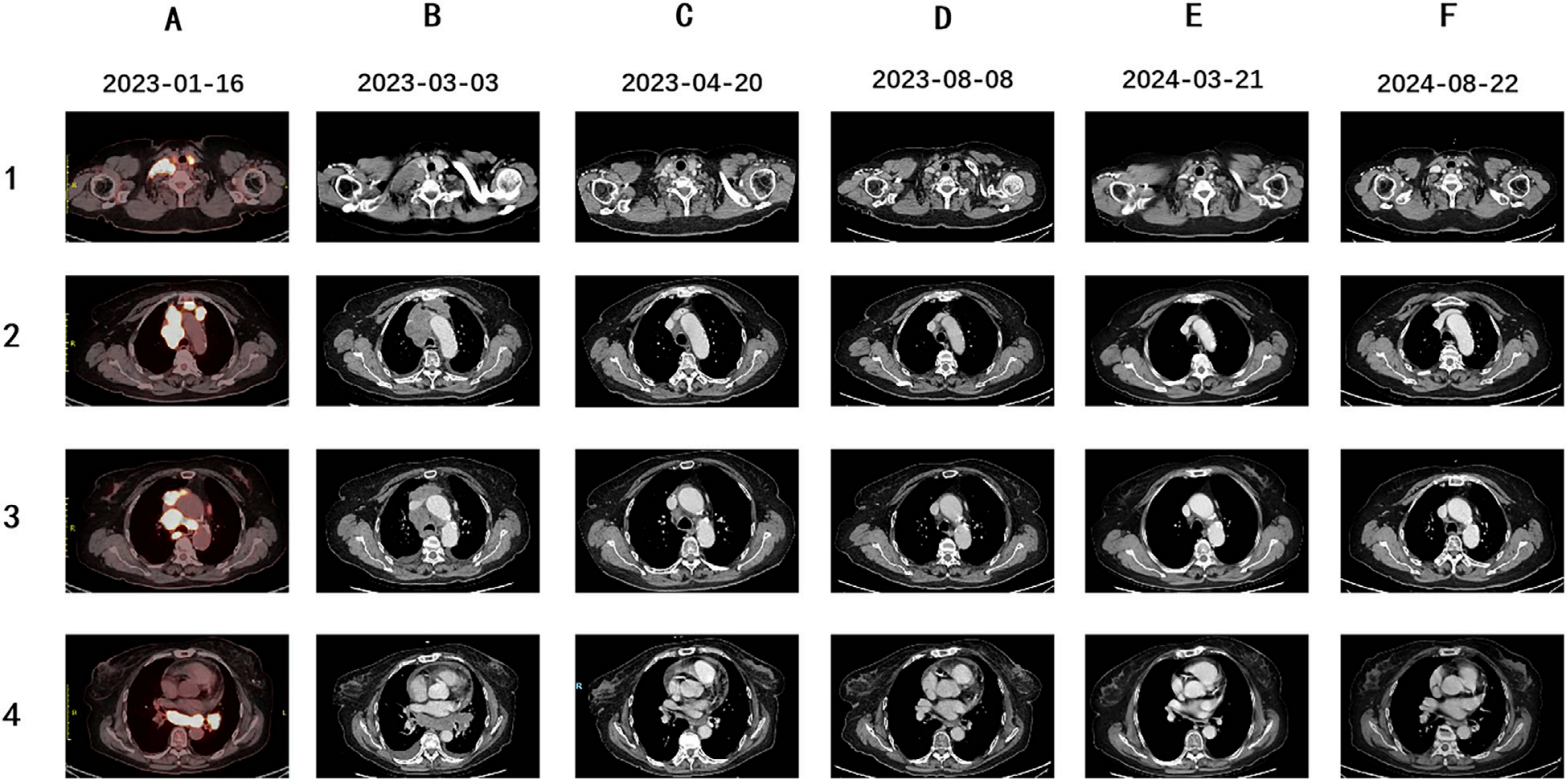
PET-CT and follow-up Enhanced CT images of this patient. (A) The patient was diagnosed as ES-SCLC. Pre-treatment PET-CT scan suggested enlarged lymph nodes (LNs) in the right supraclavicular and infraclavicular fossa, mediastinal areas, and left hilum of the lung, with increased fluorodeoxyglucose uptake level, indicating tumor metastasis. (B) Enhanced CT scan suggested enlarged area of the initial metastatic lesions in the right supraclavicular and infraclavicular fossa, mediastinal areas, and left hilum of the lung after 3 weeks of crizotinib treatment. (C) Enhanced CT evaluation revealed the significant shrinkage of multiple LNs after two courses of Etoposide, Cisplatin and Sapolizumab treatment. (D–F) During the therapeutic period of Sapolizumab as maintenance therapy, regular enhanced CT scans suggested stable disease.
